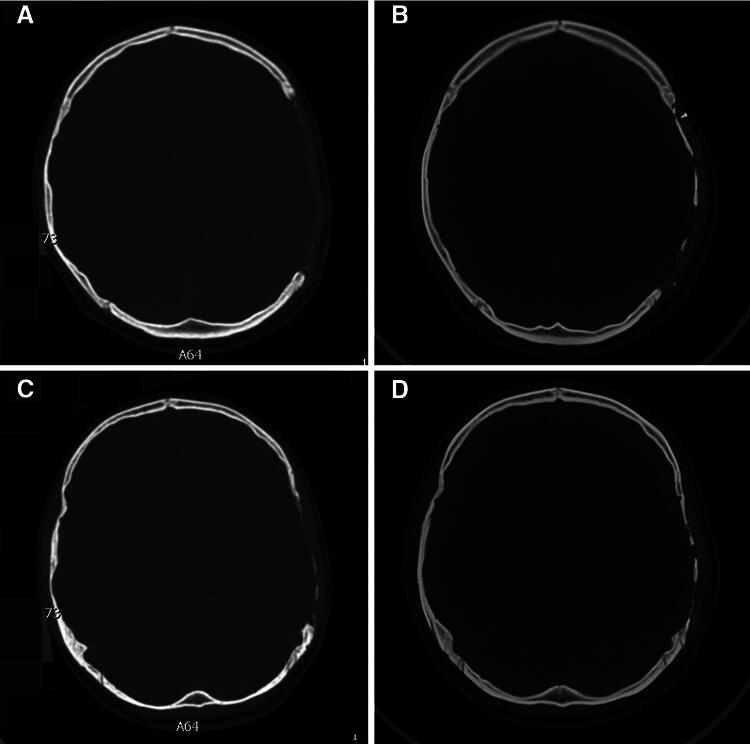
CT images of two typical patients with osteoconduction in the outer layer of the dura mater below the PEEK implant. (A, C) Preoperative CT image. (B, D) Follow-up CT image. Patient 1: A, B; patient 2: C, D. CT, computed tomography; PEEK, polyether ether ketone.
